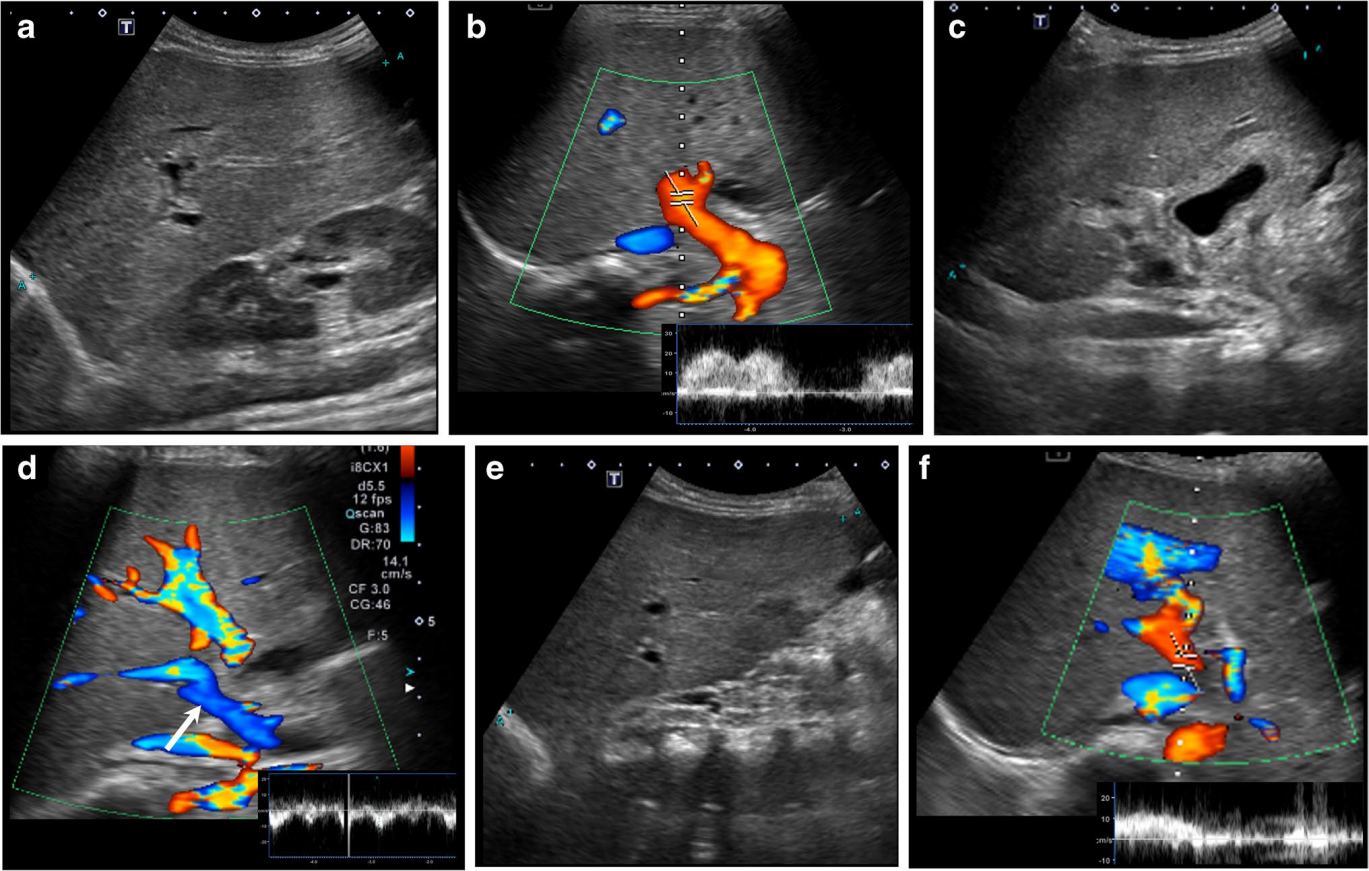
Sagittal ultrasound scans in a representative veno-occlusive disease (VOD) case.  A 6-year-old boy with acute myeloid leukemia underwent hematopoietic stem cell transplantation (HSCT). a, b Seven days after HSCT, the liver size increased to 13.4 cm (a), and the portal vein showed a typical velocity of 23.7 cm/s at the intercostal scan (b). Liver stiffness increased to 7.2 kPa and viscosity to 16.7 m/s/kHz at the intercostal scan. At that time, it did not meet the clinical diagnosis criteria for VOD. Three days later, the patient was clinically diagnosed with moderate veno-occlusive disease and treated with defibrotide. c, d Follow-up ultrasound on day 20 after HSCT revealed gallbladder wall edema (c) and reverse flow of the portal vein (*arrow* in d). Liver stiffness increased to 32.2 kPa and viscosity to 17.5 m/s/kHz. e, f A follow-up ultrasound on day 35 after HSCT showed a persistent liver size increase (e), but the portal vein recovered its flow direction and velocity (f). Liver stiffness decreased to 7.0 kPa, and viscosity remained at 16.9 m/s/kHz
